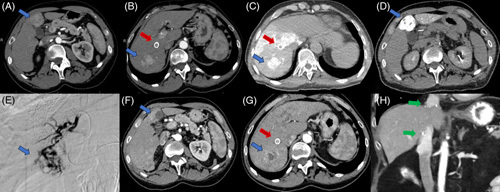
Patient with HCV cirrhosis and portal hypertension status post-TIPS (red arrow) presented with intermediate-stage HCC (blue arrows). (A) Segment IVB tumor measures 3.5 × 3.4 cm. (B) Segment VII tumor measures 3.9 × 3.0 cm. (C and D) Intraoperative hepatic arteriography–directed CT demonstrated planned radioembolization coverage with a target dose of 276 and 251 Gy, respectively. (E) Catheter angiogram selecting segment IVB tumor. (F and G) Postradioembolization arterial phase CT shows significantly decreased enhancement of target tumors. (H) Patient received liver transplant 4 months after radioembolization with follow-up CT 13 months post-transplant (green arrows indicate surgical anastomosis).
